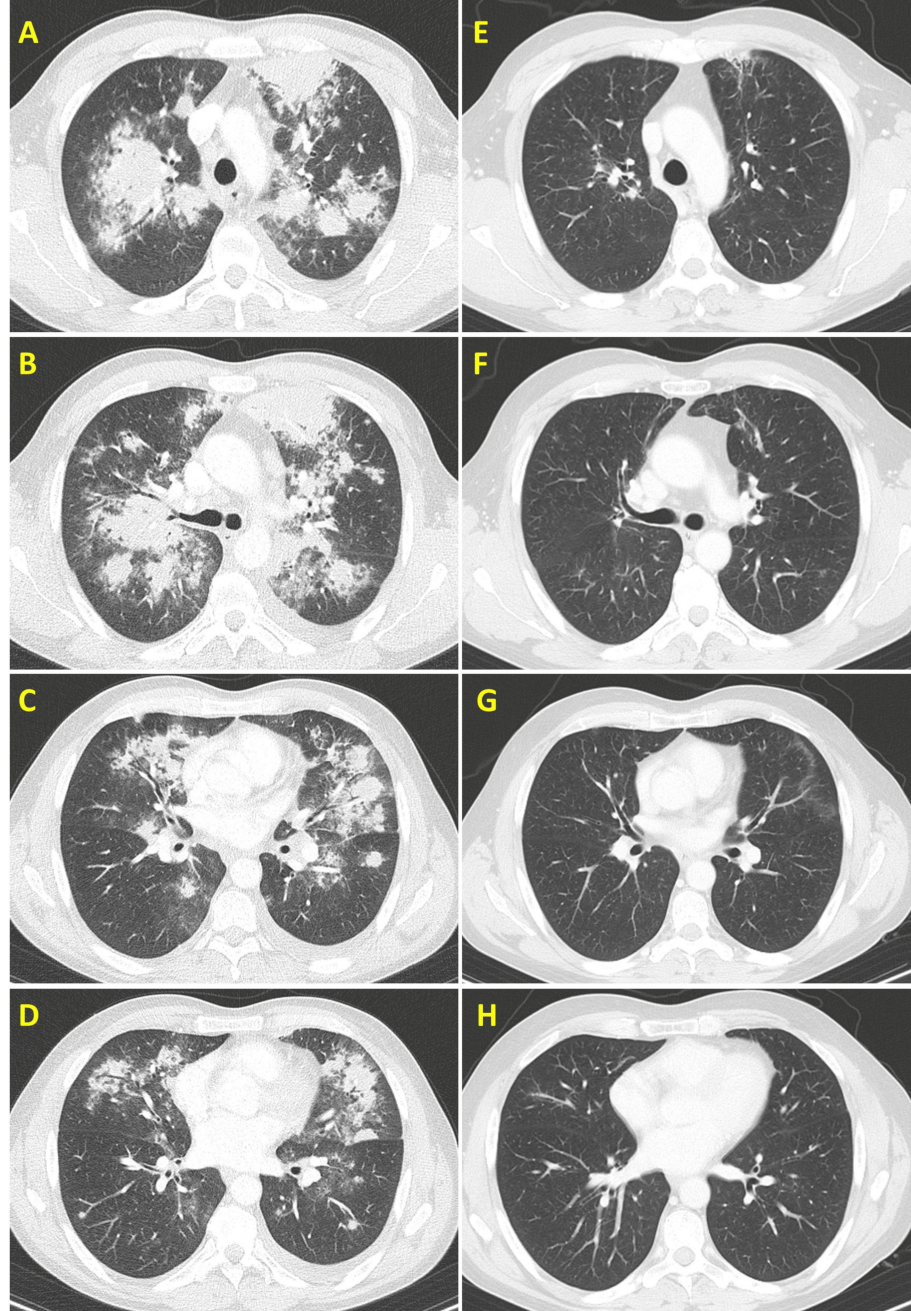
Lung involvement as shown in the emergency department CT scan (A-D) and after two years of treatment (E-H). Note the multiple lung nodules surrounded by ground-glass opacities, suggestive of alveolar hemorrhage. Comparative images show radiological improvement after induction of remission (A versus E, B versus F, C versus G, and D versus H).
